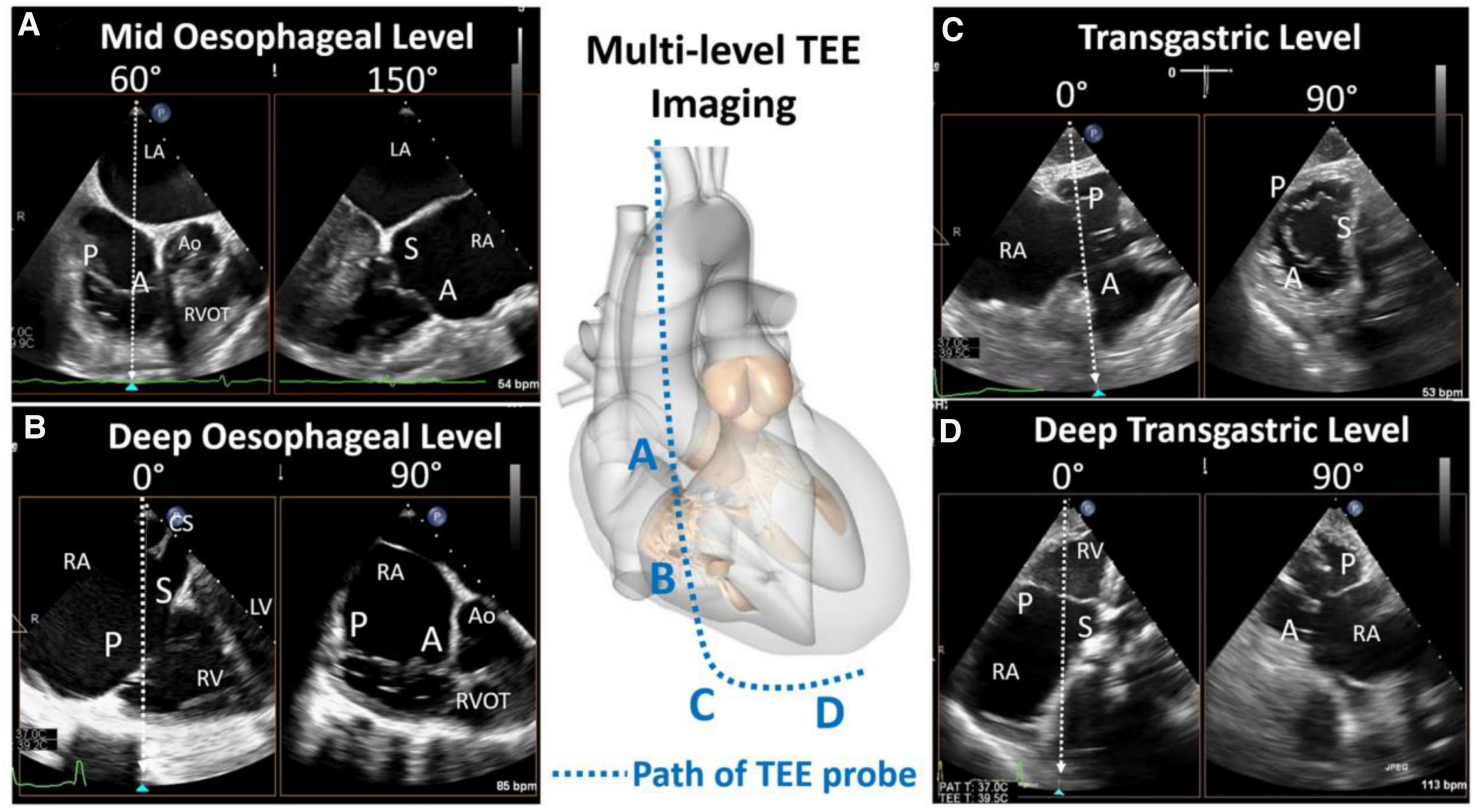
Transesophageal echocardiography imaging levels (reproduced with permission from (32)). Comprehensive tricuspid assessment requires imaging from multiple esophageal and gastric windows: (A) mid esophageal level, (B) deep esophageal level, (C) transgastric, and (D) deep transgastric. A, anterior leaflet; Ao, aorta; LA, left atrium; LV, left ventricle; P, posterior leaflet; RA, right atrium; RV, right ventricle; RVOT, right ventricular outflow tract; S, septal leaflet.
